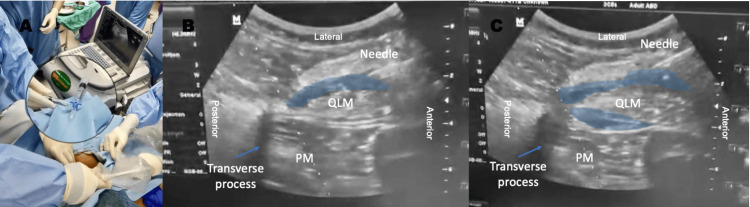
Ultrasound-guided bilateral quadratus lumborum block (QLB) and sonogram [We could not store and export the sonogram due to a faulty ultrasound storage system- pictures were taken by an amateur colleague] A. Before the local anesthetic (LA) deposit, the fascial plane was hydro-located and hydro-dissected with saline with the help of an assistant using two syringes (LA solution, 10ml, and saline, 3ml) and a three-way tap connector connecting to the Stimuplex needle (inset). B. Lateral QLB: the deposition of LA on the lateral side of the QL muscle after the needle tip penetrates the transversus abdominis muscle aponeurosis. Although LA was deposited in the lateral aspect of QL muscle, a sonogram showed LA tracking to the posterolateral aspect of QL muscle in the thoracolumbar fascia between QL muscle and paraspinal muscles. C. Anterior QLB was also performed using the same needling trajectory (anterior-to-posterior). LA was deposited in between the QL and psoas muscles. Our ultimate goal was to ensure adequate LA spread within the thoracolumbar fascial plane to improve the success of the block. Blue shade: Local anesthetic deposition; QLM: Quadratus lumborum muscle; PM: Psoas muscle
